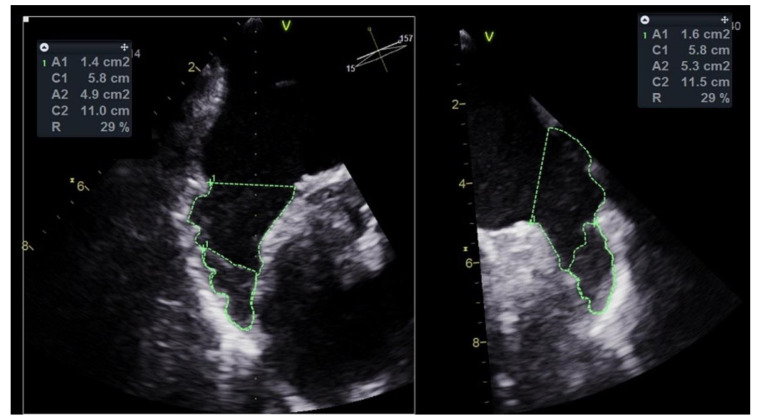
Measurement of percentage of left atrial appendage volume occupied by thrombus in transesophageal echocardiography (TEE) (Supplementary Video S1). A1 and A2, Areas 1 and 2; C1 and C2, Circumferences 1 and 2; R, ratio A1/A2.
